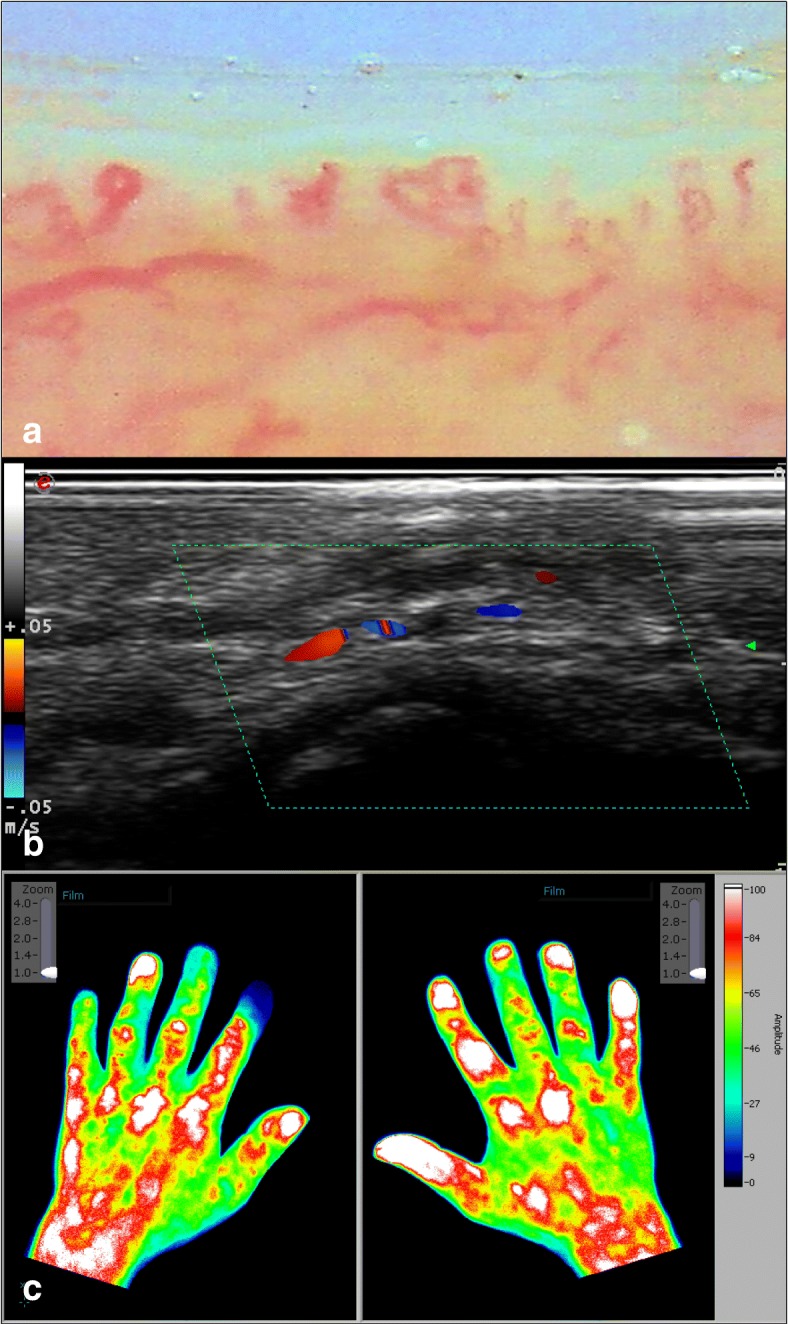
Exemplary depiction of the three imaging techniques: a Nailfold Capillaroscopy of a patient with diffuse SSc; the last capillary line is disorganized, the capillaries are rarefied (late pattern). b Colour Doppler ultrasonography shows a narrowed PPDA in a patient with diffuse SSc. c Fluorescence optical imaging in a patient with diffuse SSc; the index finger of the left hand shows a missing ICG enhancement as a sign of perturbed perfusion. SSc—systemic sclerosis; PPDA—proper palmar digital artery; ICG—indocyanine green
